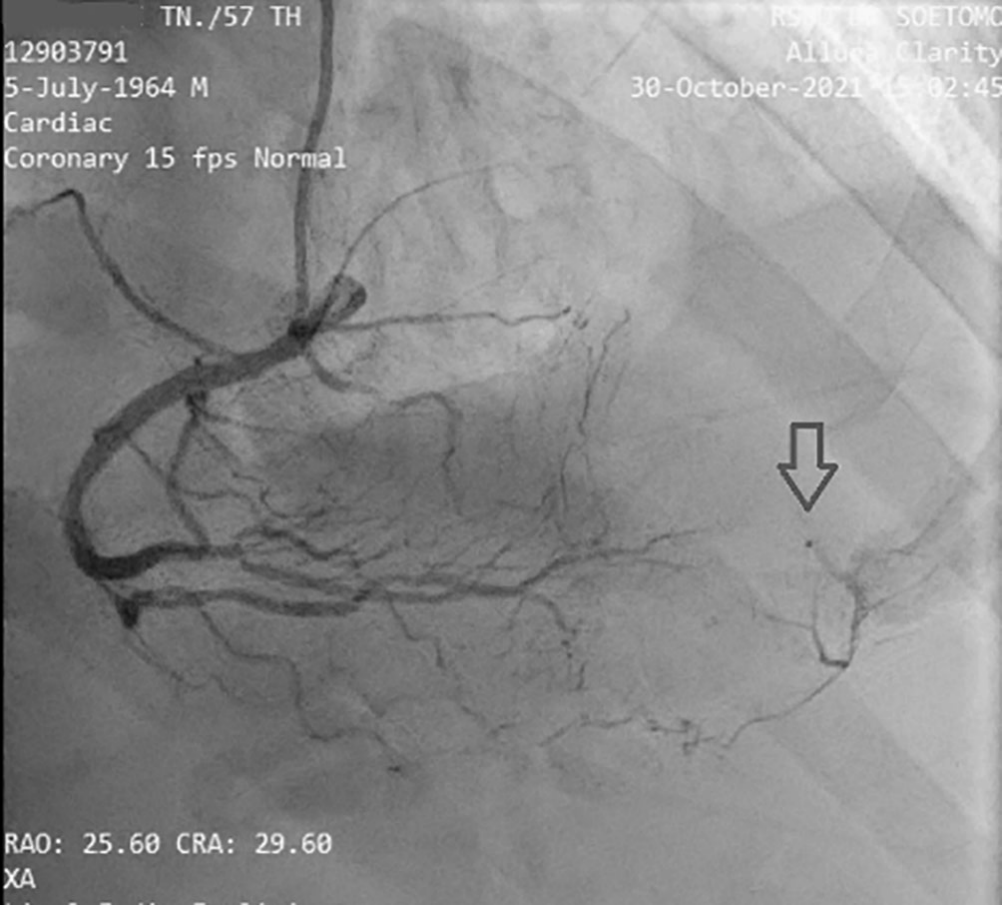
Angiography RCA projection View RAO, CRA. It is revealed that there is grade II collateral from distal right coronary artery to distal left anterior descending coronary artery. (Notes: RAO CRA = right anterior oblique + cranial view, a radiographic projection).
